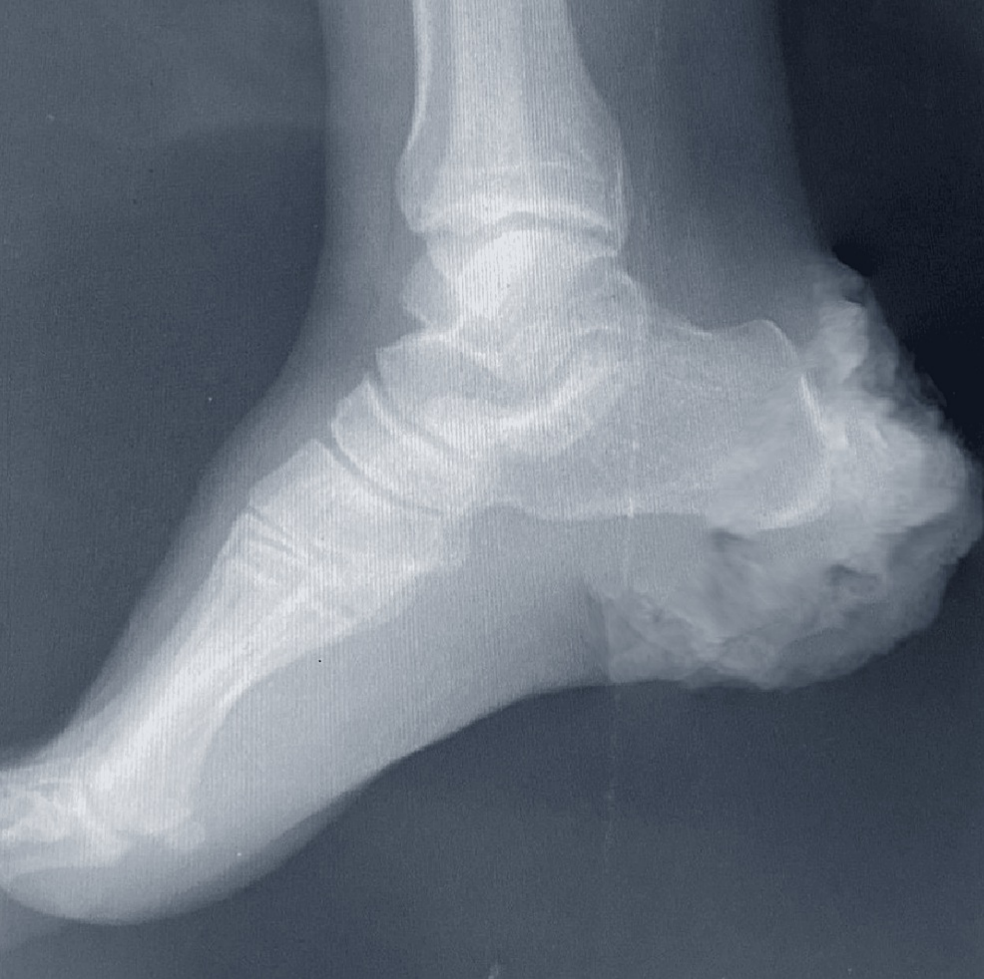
X-ray imaging of the right heel, with cutaneous lesion visible and no evidence of bone invasion.
